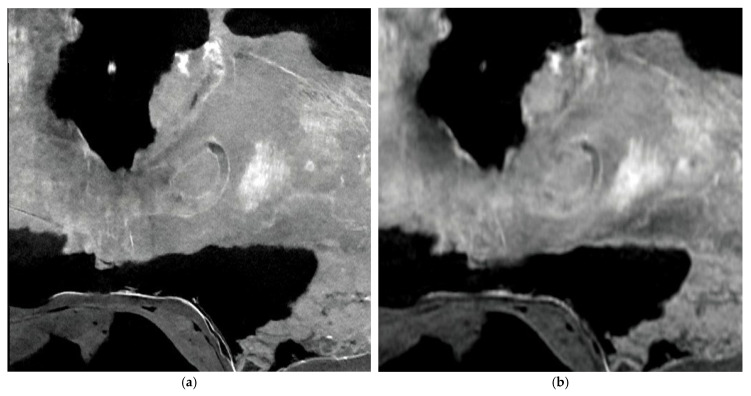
Cross-sections of the *Camarasaurus* temporal bone imaged with PCD-CT (a) and EID-CT (b).
